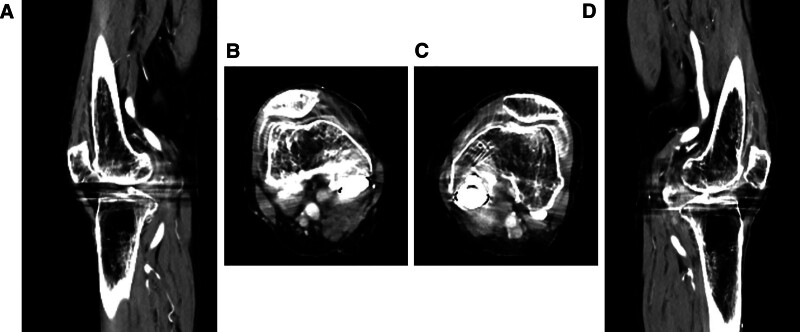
(A) The sagittal images of contrast-enhanced CT for the right knee joint after steroid administration. (B) The axial images of contrast-enhanced CT for the right knee joint after steroid administration. (C) The axial images of contrast-enhanced CT for the left knee joint after steroid administration. (D) The sagittal images of contrast-enhanced CT for the left knee joint after steroid administration.
